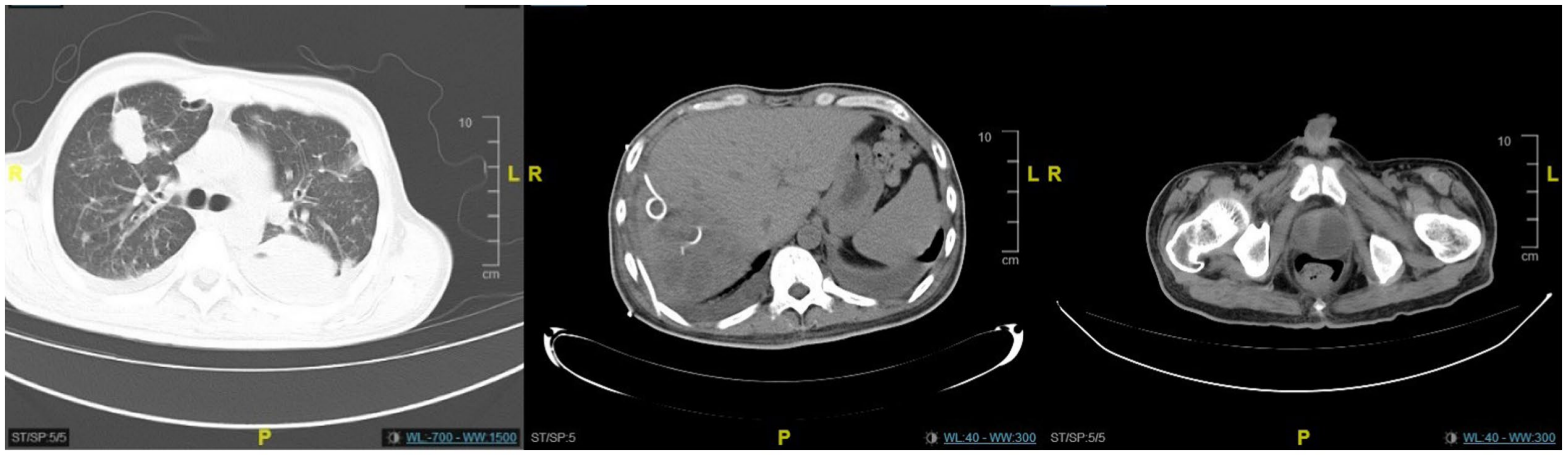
The CT scan revealed an abscess in the right lobe of the liver with multiple areas of pneumocephalus, an enlarged and distended prostate containing low-density lesions, and pelvic effusion. Bronchiectasis with infection was observed in the right lower lobe of the lung. Bilateral pleural effusion was present, along with distension of the lower lobes in both lungs.
